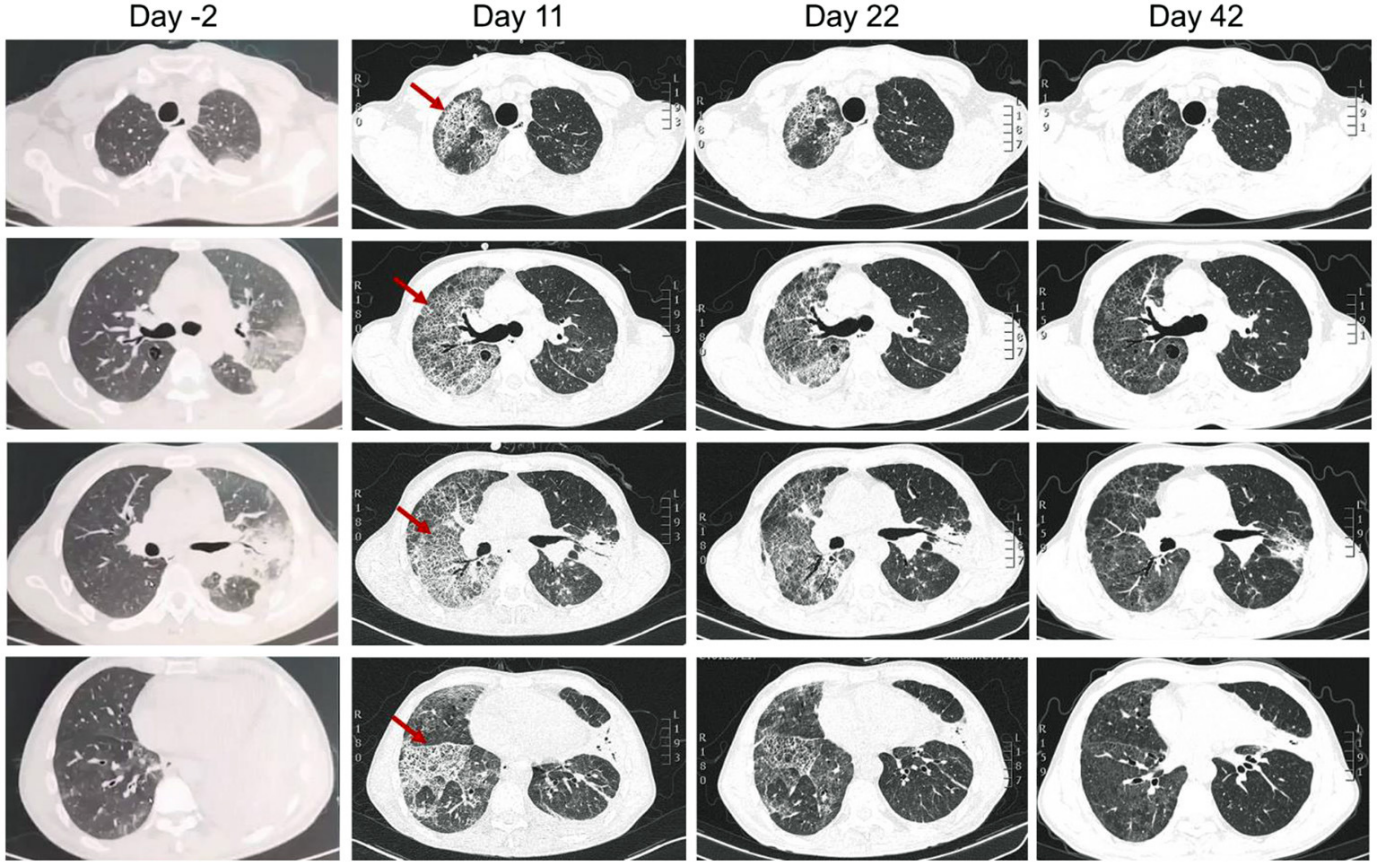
Chest computed tomography of the patient on 2 days before admission (day −2), and day 11, 22, and 42 (20 days after discharge) after admitted to our hospital. Arrows indicated the right lung developed interstitial infiltrates on day 11.
